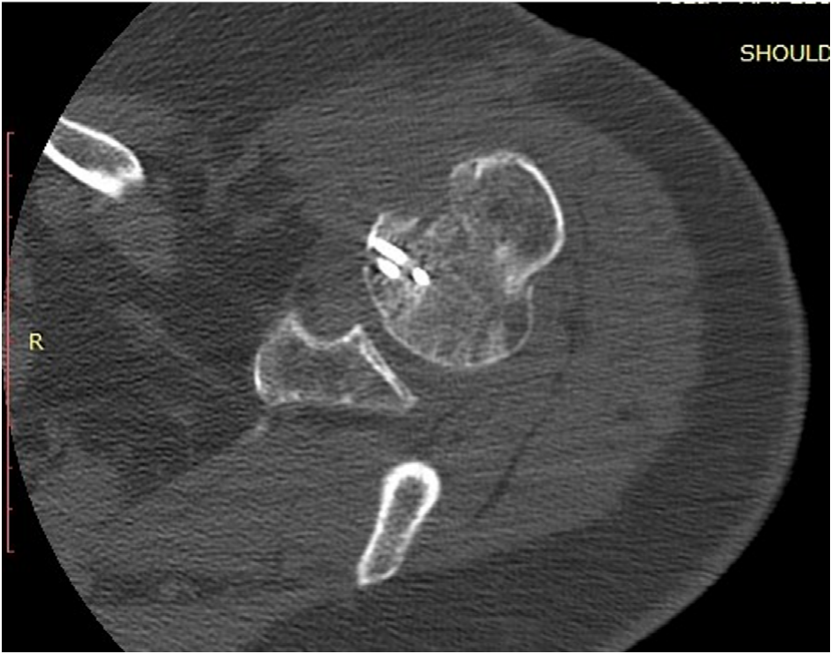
2nd patient: Postoperative CT scan of Left shoulder, after modified McLaughlin procedure and reverse Bankart repair. Humeral head is centered in the glenoid. A gap after lesser tubercle transfer is obvious-left behind. The suture anchor used for the reverse Bankart repair is not visible in CT sections.
